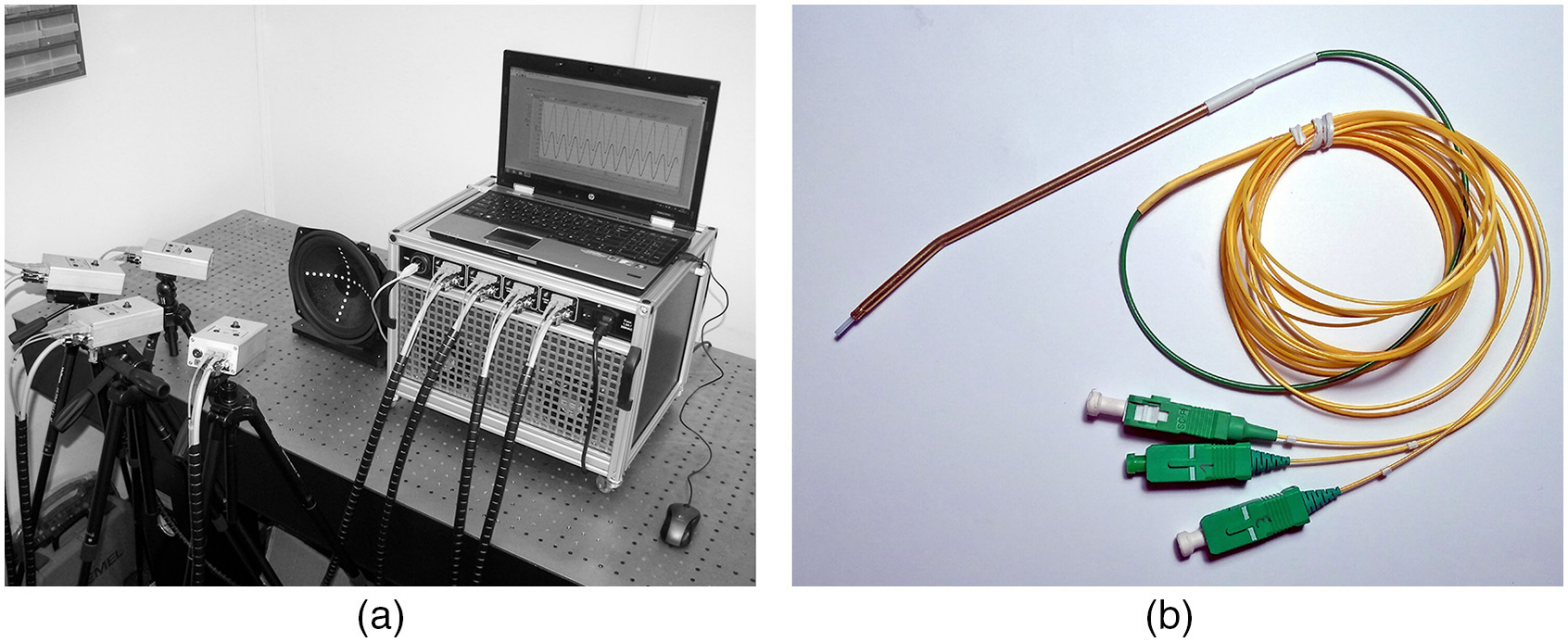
(a) The four channel laser-fiber Doppler vibrometer^47^ and (b) the handheld probe. The probe, consisting of a ceramic ferrule with the three optical fibers mounted inside a metal shielding sleeve, was connected to one of the four channels of the laser-fiber vibrometer. Two optical fibers are for the forward and return paths of the 1550 nm laser, and one is for the red navigation spot.
